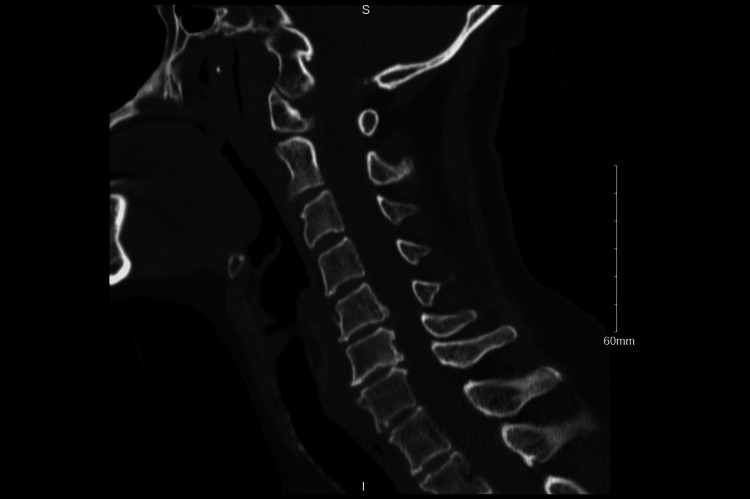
CT spine cervical without contrast No acute cervical spine fracture or traumatic malalignment.
